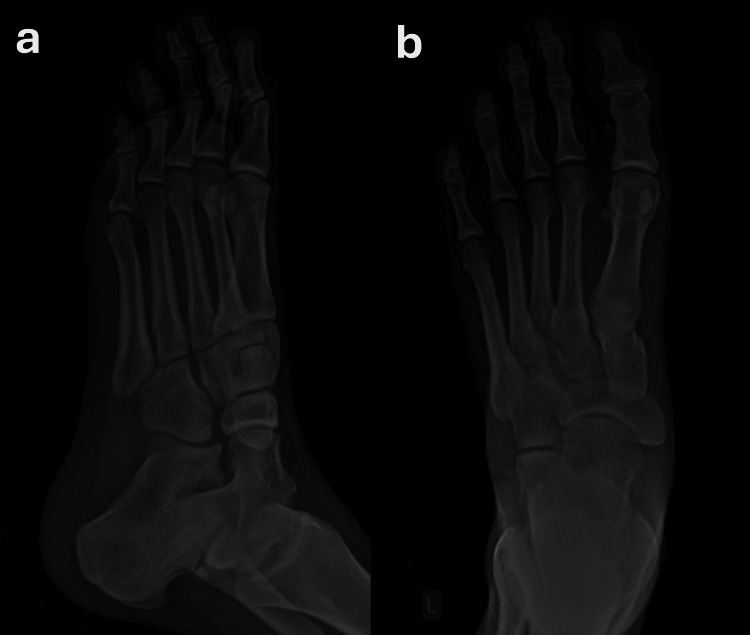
X-ray of the left foot - (a) oblique view; (b) plane view
